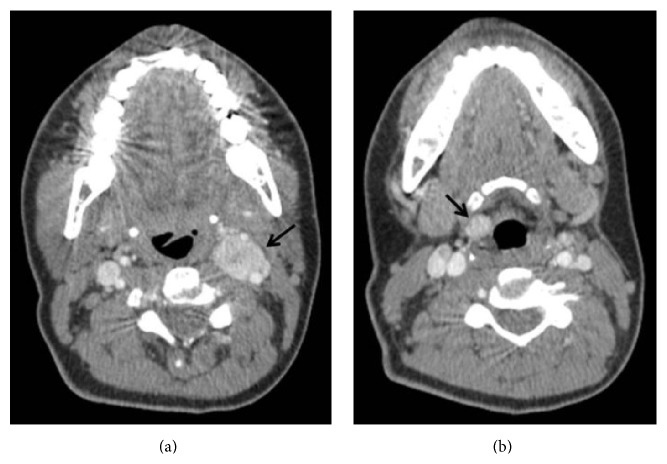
Axial contrast enhanced computed tomography demonstrates vascular mass (arrow) at left carotid bifurcation (a). Right supraglottic, well defined laryngeal mass (arrow) is seen in lower level contrast enhanced computed tomography image (b).
